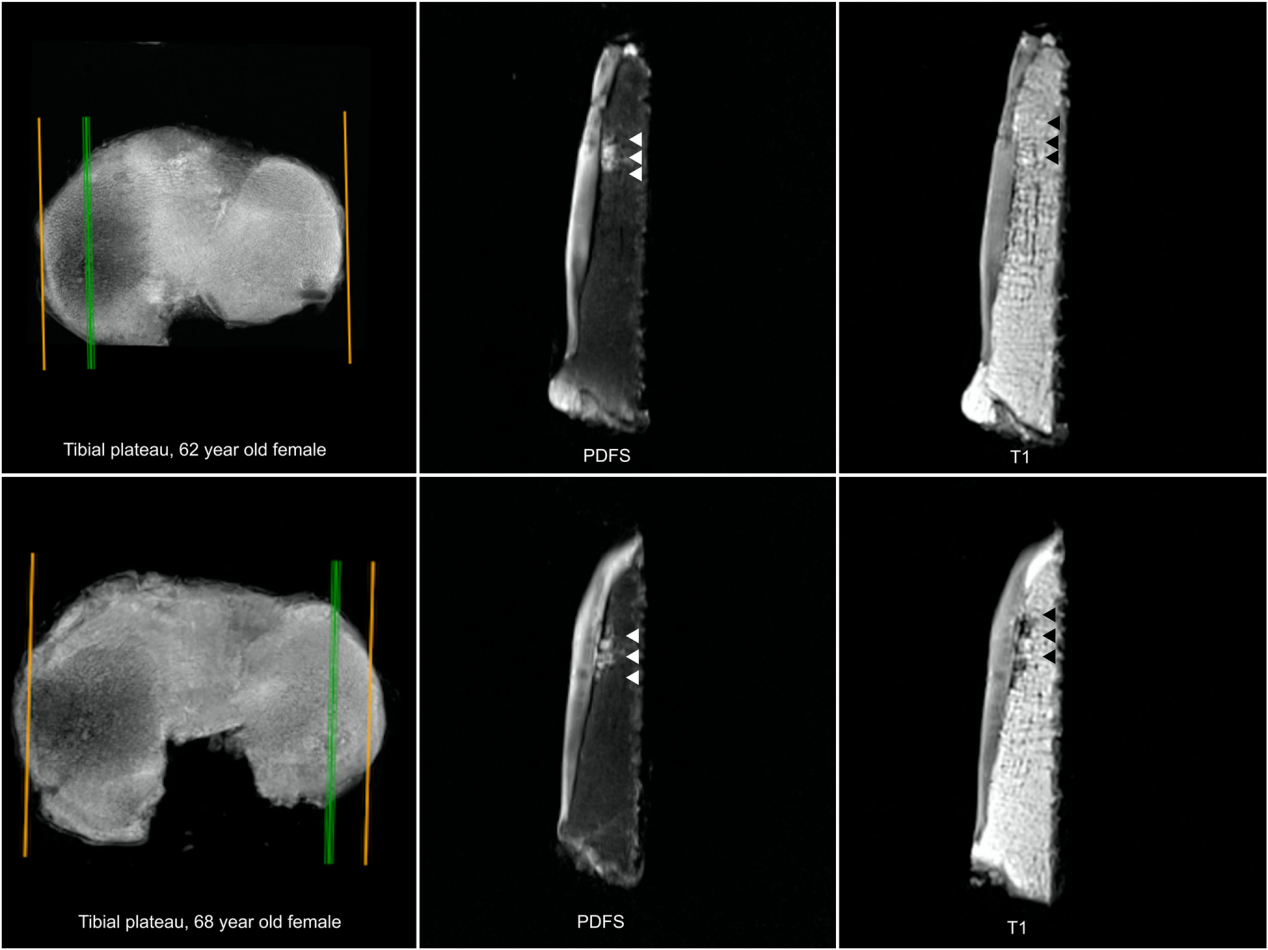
Left*, *upper* and *lower panels* show tibial plateaus in the transaxial plane. *Right*, *green line* is the sagittal slice. *Upper panel*, *middle*, the signal of the bone marrow lesion (BML) is detected by fast spin-echo proton density-weighted (PDFS) sequence (*arrows* identify signal), and *right* the signal is not detected by T1-weighted sequence. *Lower panel*, *middle*, the signal of the BML is detected by PDFS sequence (*arrows* identify signal), and *right* the signal is detected by T1-weighted sequence
